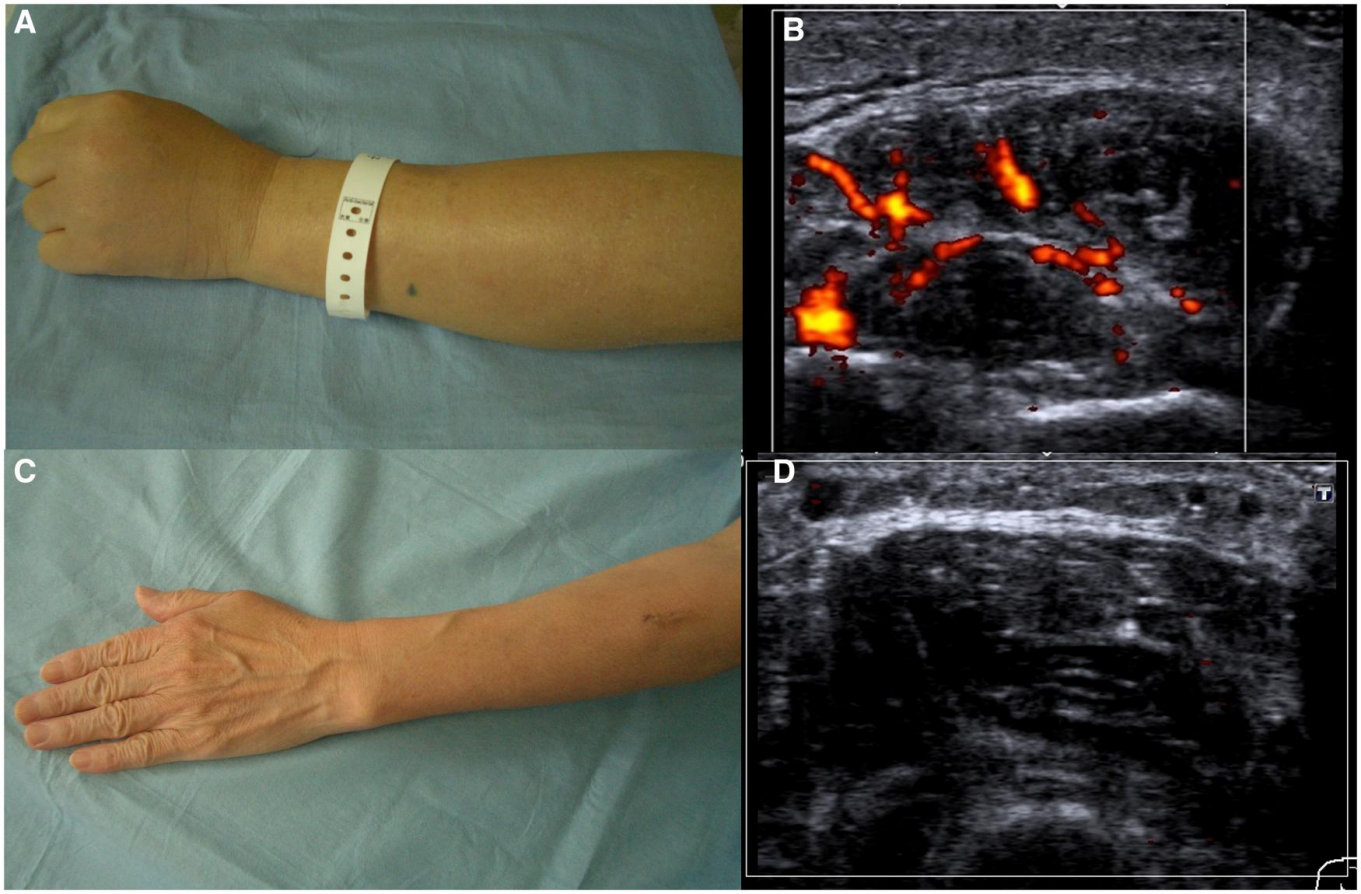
Power Doppler ultrasonography findings of eosinophilic fasciitis. (A) Swelling in the left forearm. (B) Abnormal Doppler signals in the left forearm fascia. (C) No swelling was noted in the left forearm after treatment. (D) No abnormal Doppler signals were noted in the left forearm after treatment
